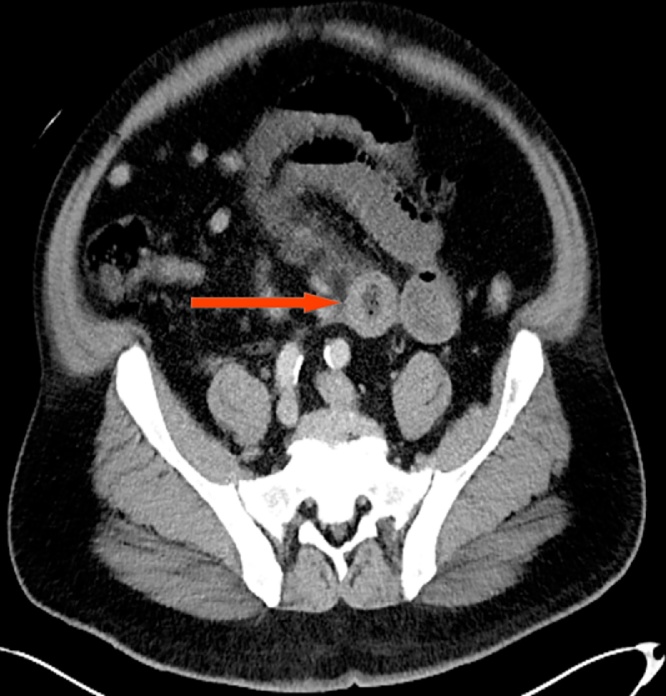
Computed Tomography of abdomen axial view showing dilated small bowels (target sign) (red arrow).
